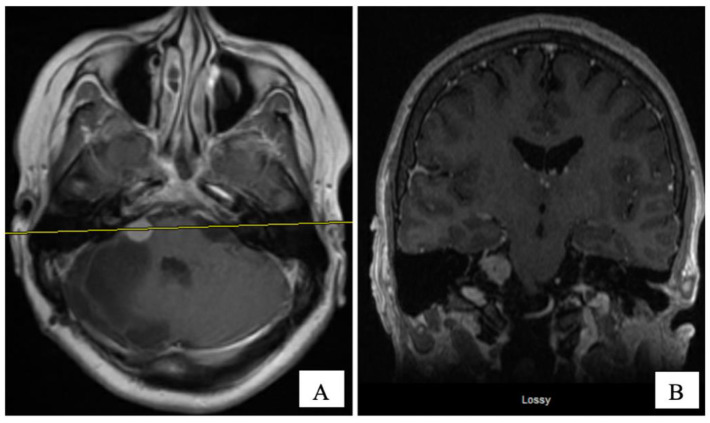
Post-gadolinium T1-W MRI at four years post CK, on axial (A) and coronal (B) planes. The CPA tumour shrank to 12 × 12 mm.
